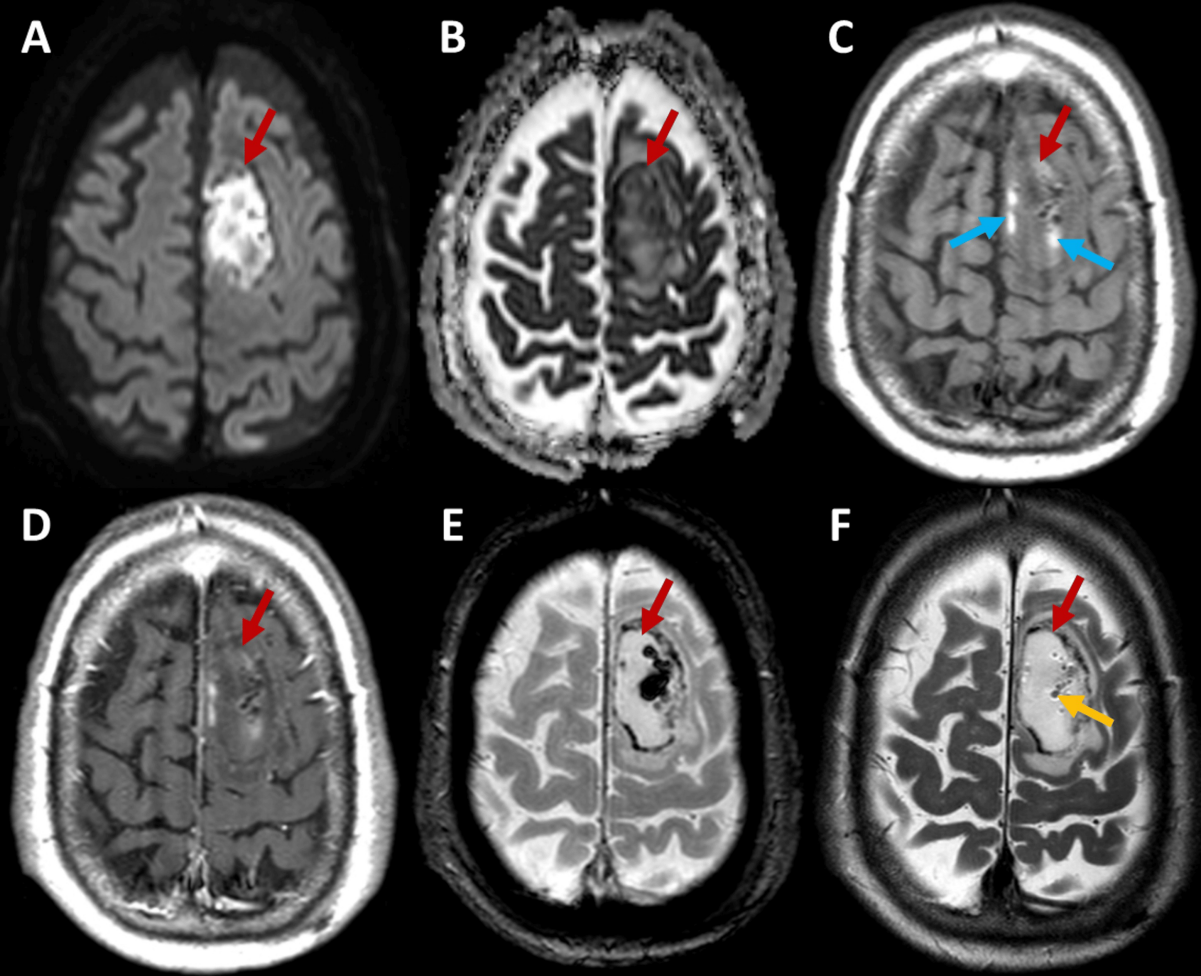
Brain MRI images in axial planes (A-F) demonstrate an ovoid lesion in the left frontal lobe (red arrows) with a gaseous component (yellow arrow). The lesion shows significant diffusion restriction and peripheral regions suggestive of a hemorrhagic component (blue arrows). No anomalous contrast uptake is observed.
